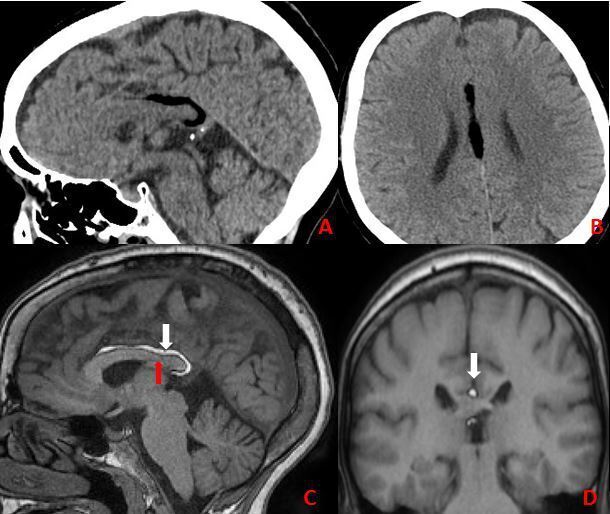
A, B: Brain CT sagittal and axial plans shows well-defined low-density in the pericallosal area and the interhemispheric fissure. C, D: Pericallosal curvilinear lipoma. Sagittal and coronal T1W MRI demonstrates a hyperintense lesion (white arrows) superior and posterior to the corpus callosum with mild dysgenesis of corpus callosum (red arrow).
